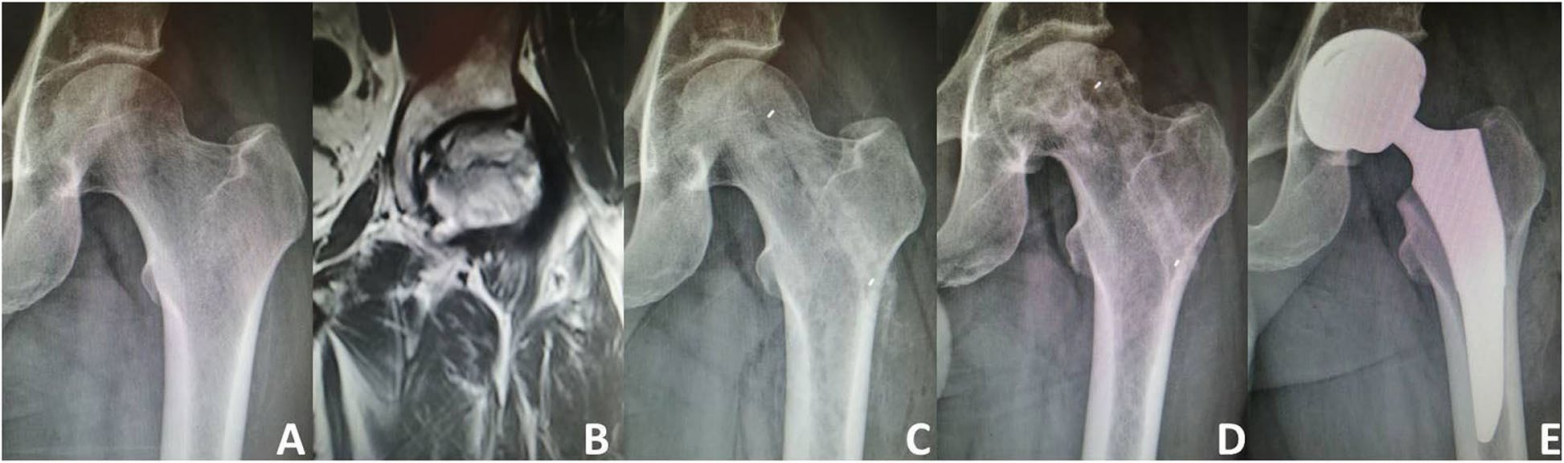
Representative case of treatment failure A Preoperative radiograph. B Preoperative MRI. C Radiograph taken on postoperative day 3. D Radiographs taken 1 year after surgery showing severe collapse of the femoral head. E The operation failed and a hip replacement was eventually performed
